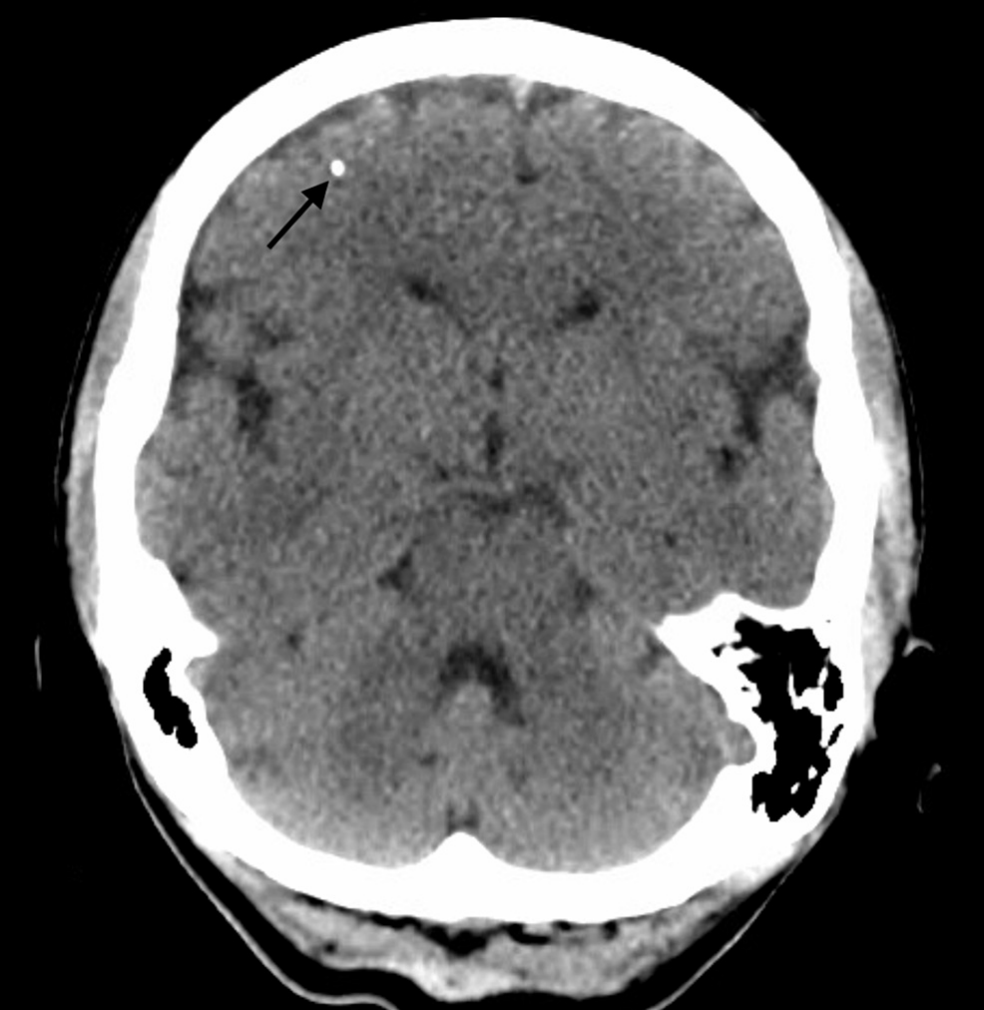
Head CT without contrast showing 1 mm calcification (arrow) in the right frontal lobe at the grey-white matter junction.
